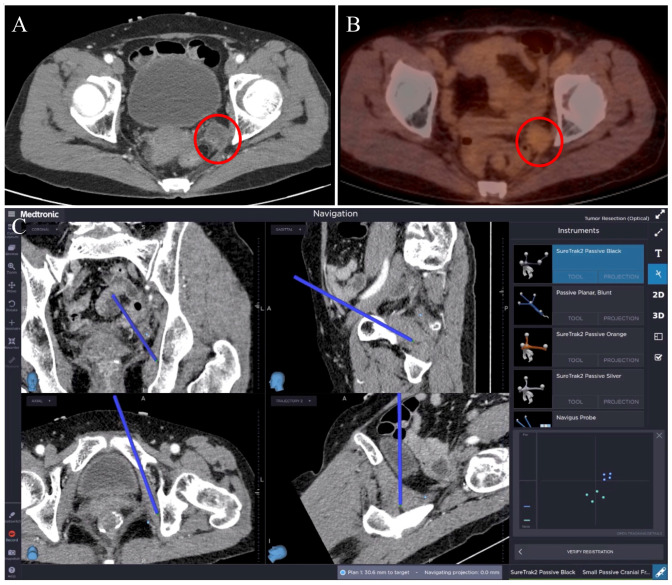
(A) CT scan and (B) positron emission tomography showing a pelvic left lateral lesion (red circles). (C) Multiplanar real-time view on the navigation software interface of the dissection along the lateral pelvic wall. The blue straight line in each quadrant shows the instrument’s position for each plane at the inferior margin of the pelvic left lateral recurrence.
